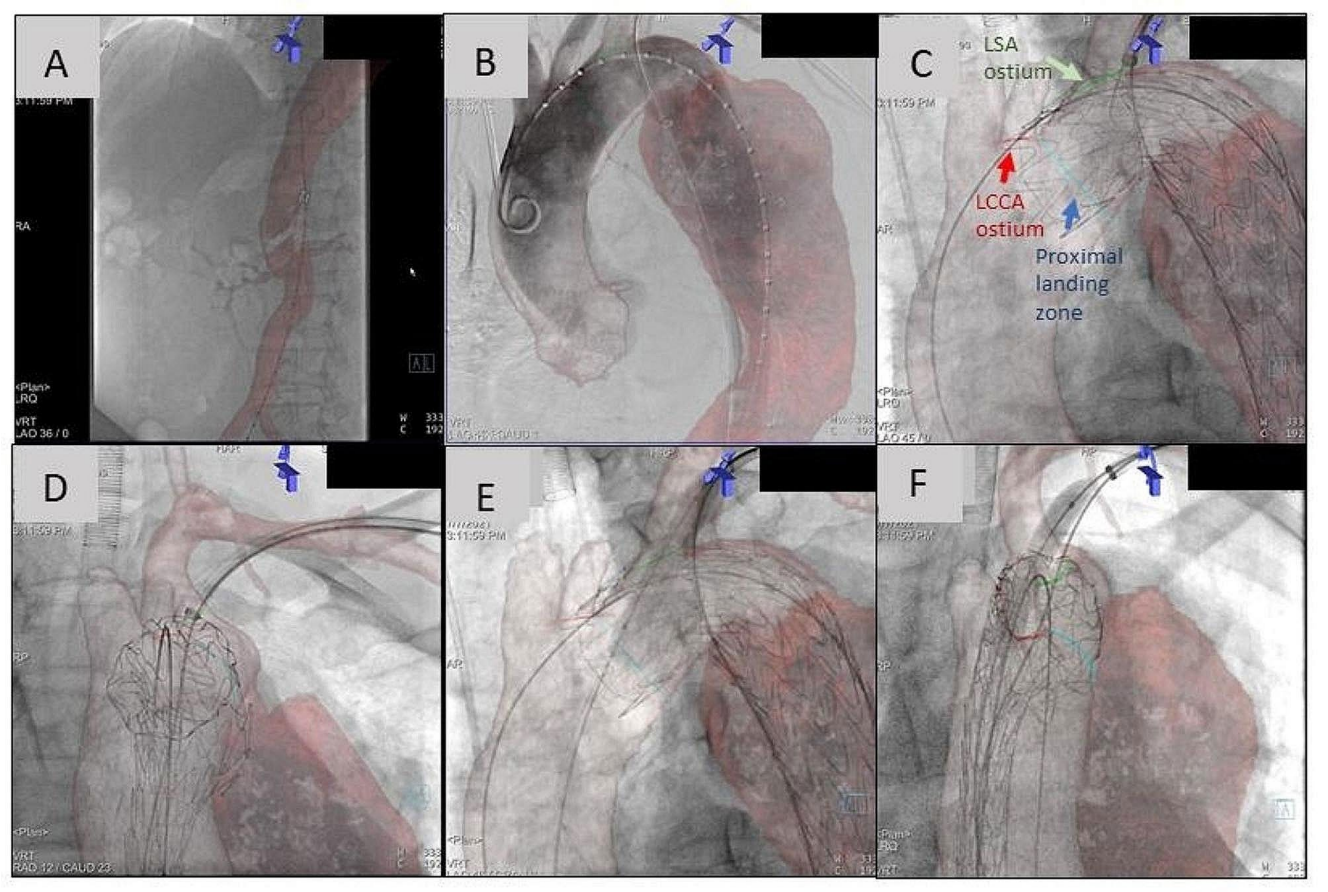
Representative case showing intraprocedural guidance under image fusion. (A) The device was introduced along the true lumen of the abdominal aorta, clearly displayed with fused CTA imaging. (B) The device reached the aortic arch with contrast injection, which confirmed the accuracy of the fused image. (C) Deployment of graft stent and perforation under image fusion guidance at an optimal working angle (arch view). Image fusion showing the centre of the LSA ostium to facilitate the accurate positioning of perforation. (D) Deployment of graft stent and perforation under image fusion guidance (en face view). Real-time three-dimensional fusion guidance reduced repeated angiographies between two common working angles: (E) predilation of balloon under image fusion guidance (arch view); (F) predilation of balloon under image fusion guidance (en face view). LSA: left subclavian artery; LCCA: left common carotid artery
